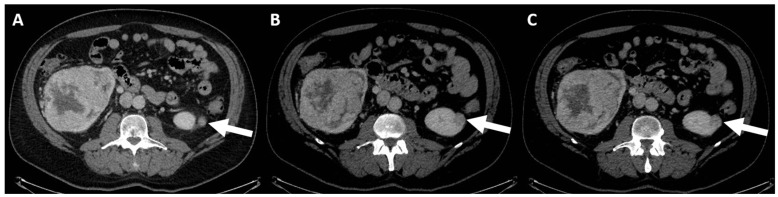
CT scan with intravenous contrast, corticomedullary phase (A–C): small left cortical enhancing renal lesion in patient with right renal tumor (arrows). The findings are consistent with Class III Bosniak.
